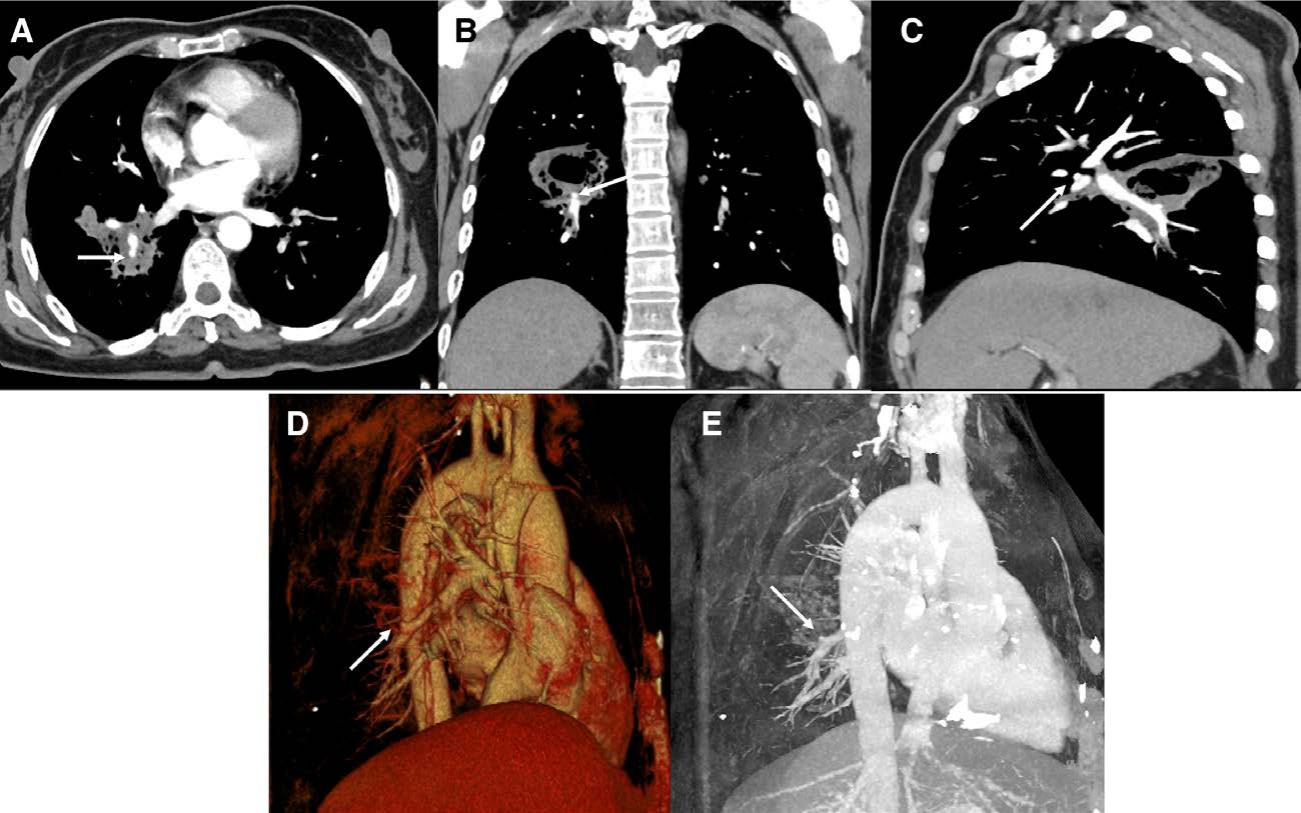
After radiotherapy and chemotherapy. Contrast-enhanced CT scan (A) shows a pseudoaneurysm (arrow) of the right lower lobe subsegmental pulmonary artery and surrounding opacity. (B) Coronal reconstructed CT image and (C) sagittal reconstructed CT image show pseudoaneurysm (arrow) encased by the tumor. Volume rendering (D) and maximum intensity projection (E) show the pseudoaneurysm (arrows) in the right lower lobe.
